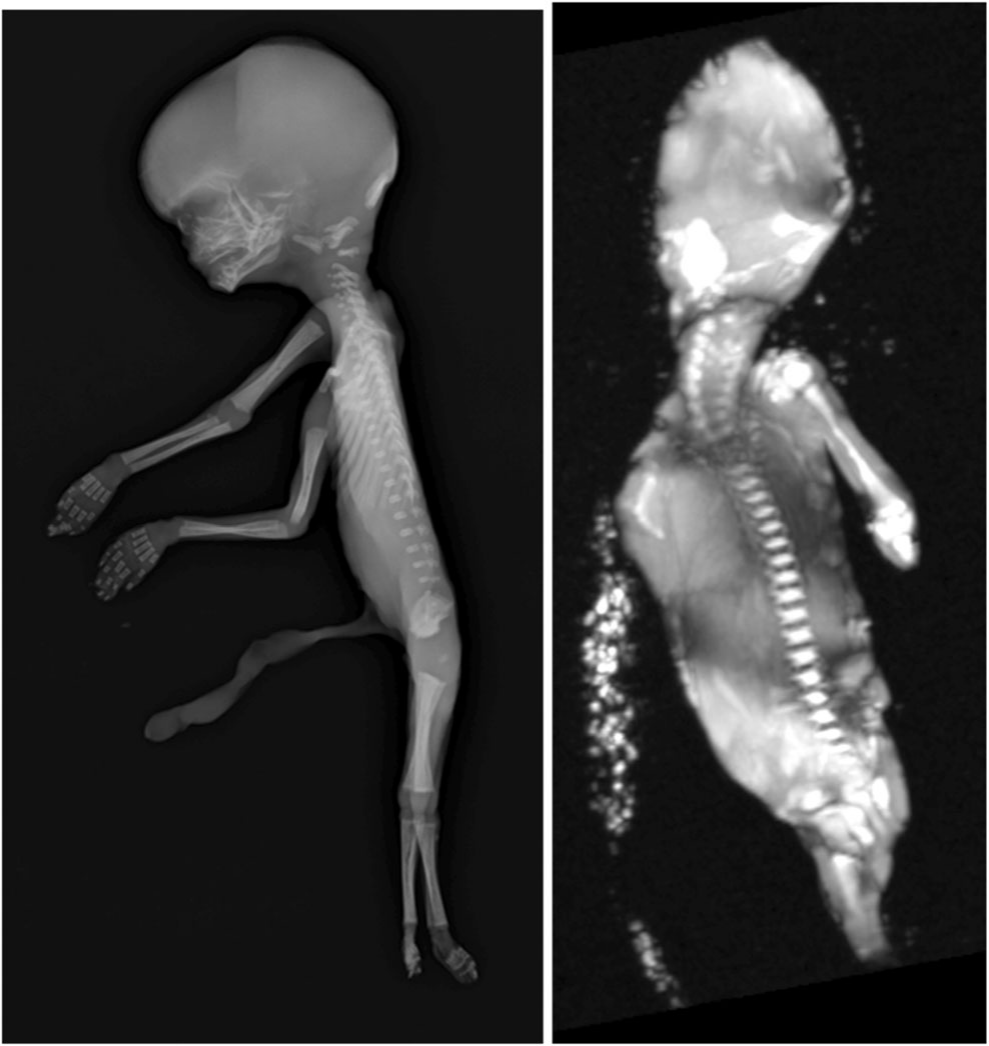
Limits of body PMMR imaging. 3D reconstruction from high resolution CISS PMMR image of a 14 week gestation (b). Skeletal radiography (a) at this gestation is often better than the highest resolution imaging at 1.5 T PMMR (b), which at low body weights is often non-diagnostic [58]
